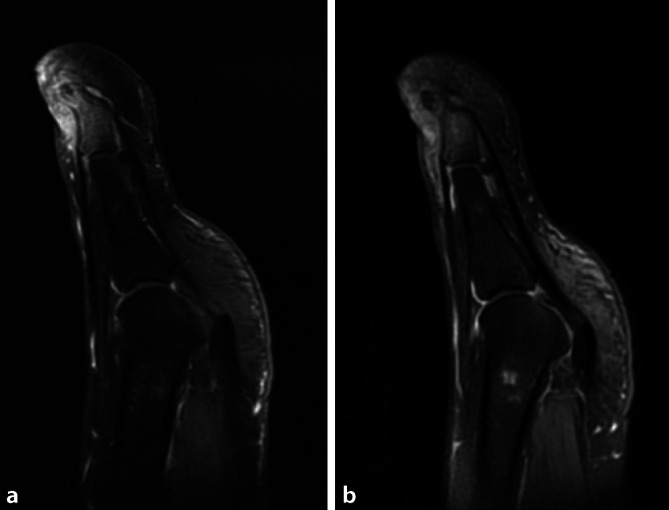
Eine interventionelle Probenentnahme war aufgrund der geringen Größe der Läsion von 7 × 4 mm und der direkt subungualen Lage nicht möglich. Die Patientin beschrieb einen hohen Leidensdruck und klagte fortwährend über eine deutlich eingeschränkte Lebensqualität mit dauerhafter Analgetikaeinnahme, eingeschränkter Gehstrecke und persistierenden Schmerzen. Ein Jahr nach Beschwerdebeginn erfolgte aufgrund der spezifischen Anamnese letztendlich eine Dünnschicht-CT (Abb. 4). Diese zeigte eine ovale Aufhellung mit zentraler punktueller Verschattung und umgebender Sklerosierung (im Sinne eines klassischen Nidus) und letztendlich den hochgradigen Verdacht auf ein Osteoidosteom.
